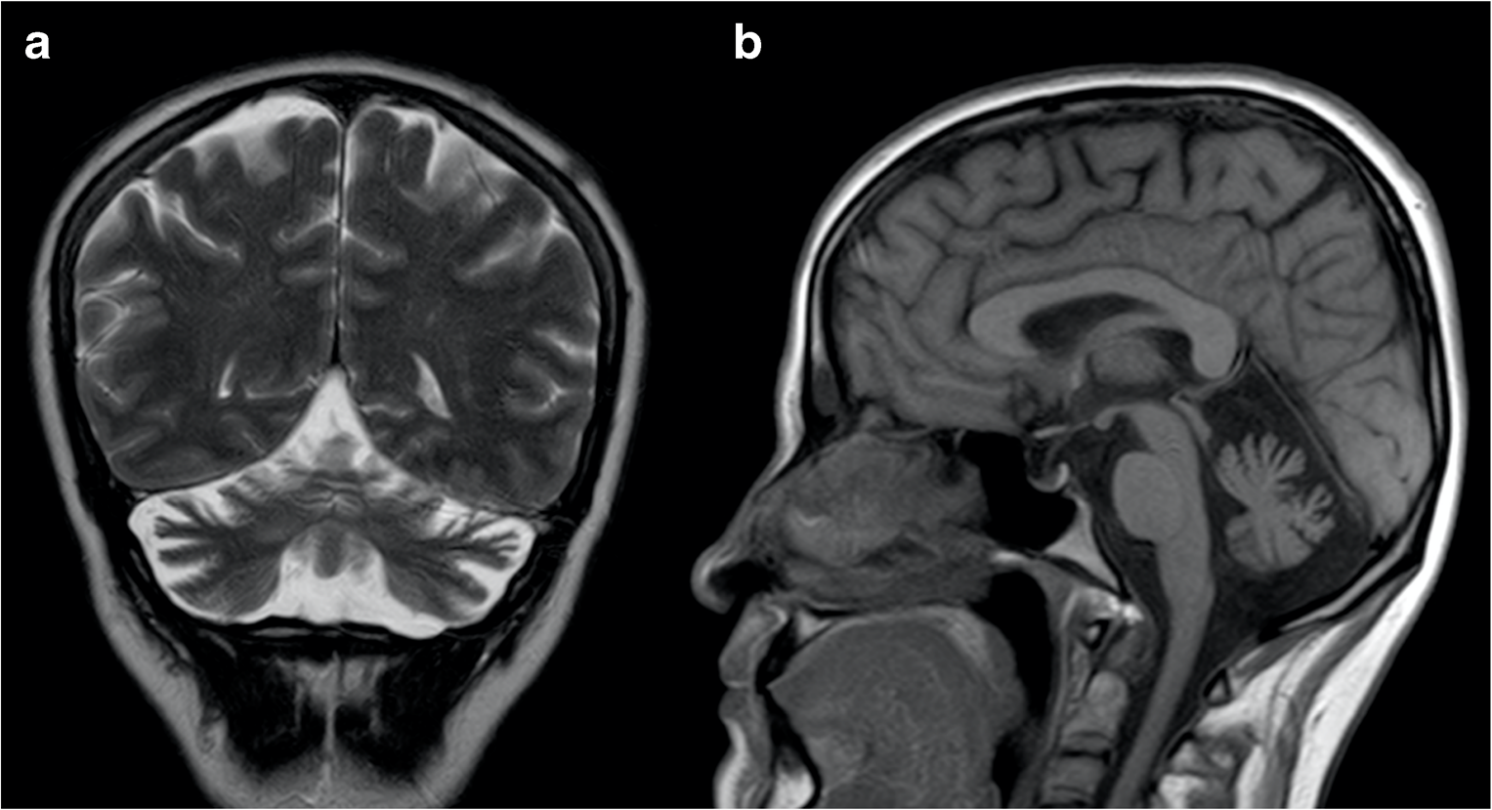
Brain MRI scan of 43-year-old male SCA8 patient. Coronal T2- (a) and sagittal T1- (b) weighted images show global cerebellar atrophy, with a relative sparing of the pons
